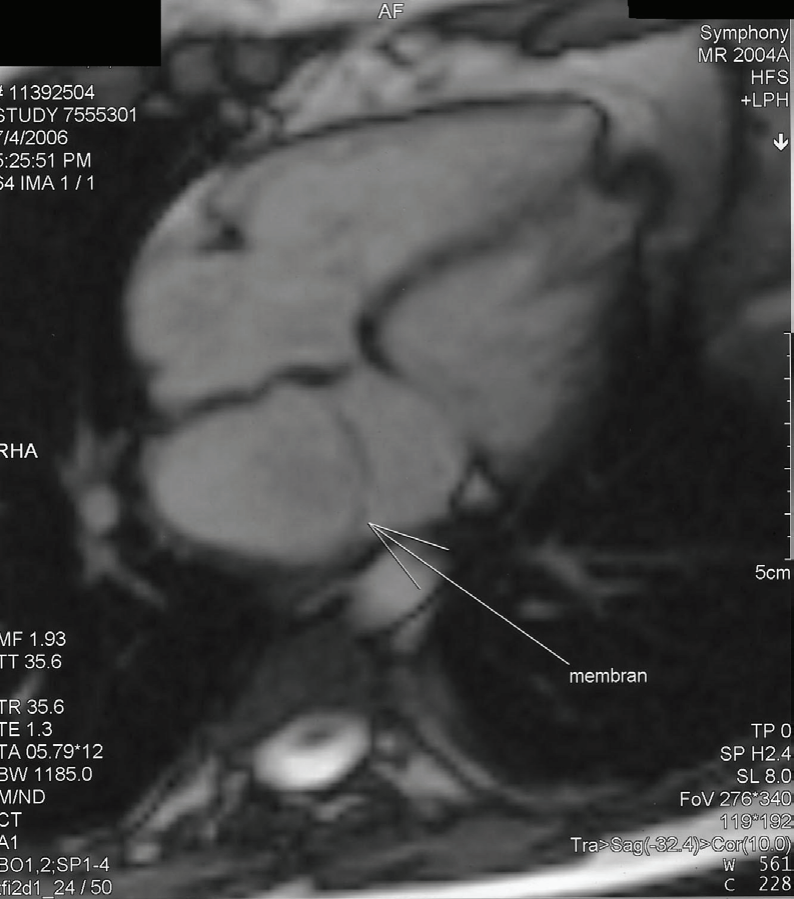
Cardiac MRI. An incomplete membranous structure, which divides the left atrium into 2 distinct chambers from the left lateral across to medial wall, has been imaged. Contrast material has been allowed to pass through this lesion.
